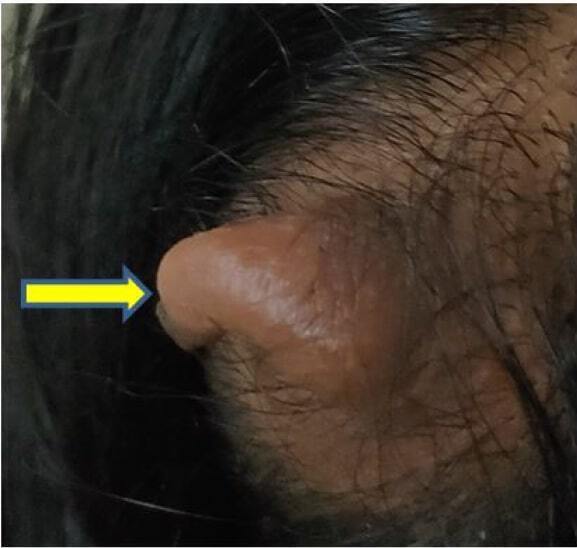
On MR imaging, a posterior interhemispheric mass lesion measuring 5.0 × 2.5 × 1.2 cm is seen posteroinferior to the superior sagittal sinus. It is hyperintense on *T*
